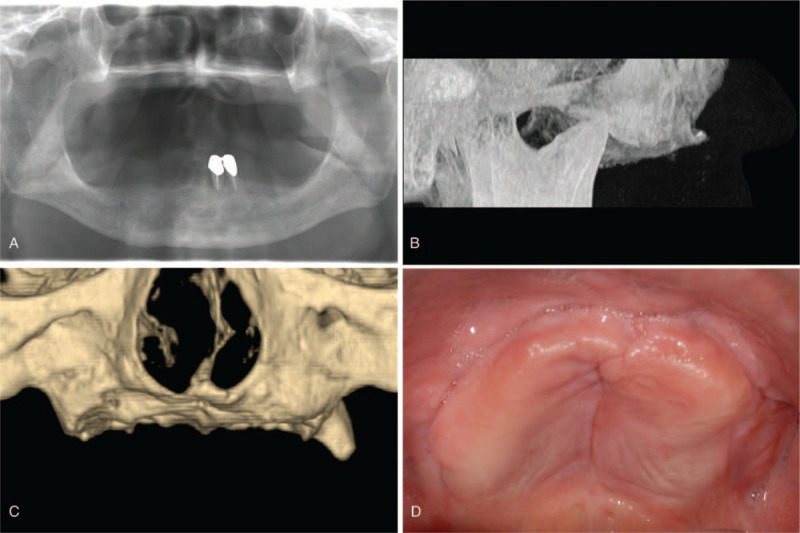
Pretreatment state. (A) Panoramic radiograph. (B) Lateral view in computed tomography. (C) Frontal view, reconstructed computed tomography image. (D) Occlusal view of the maxilla.
